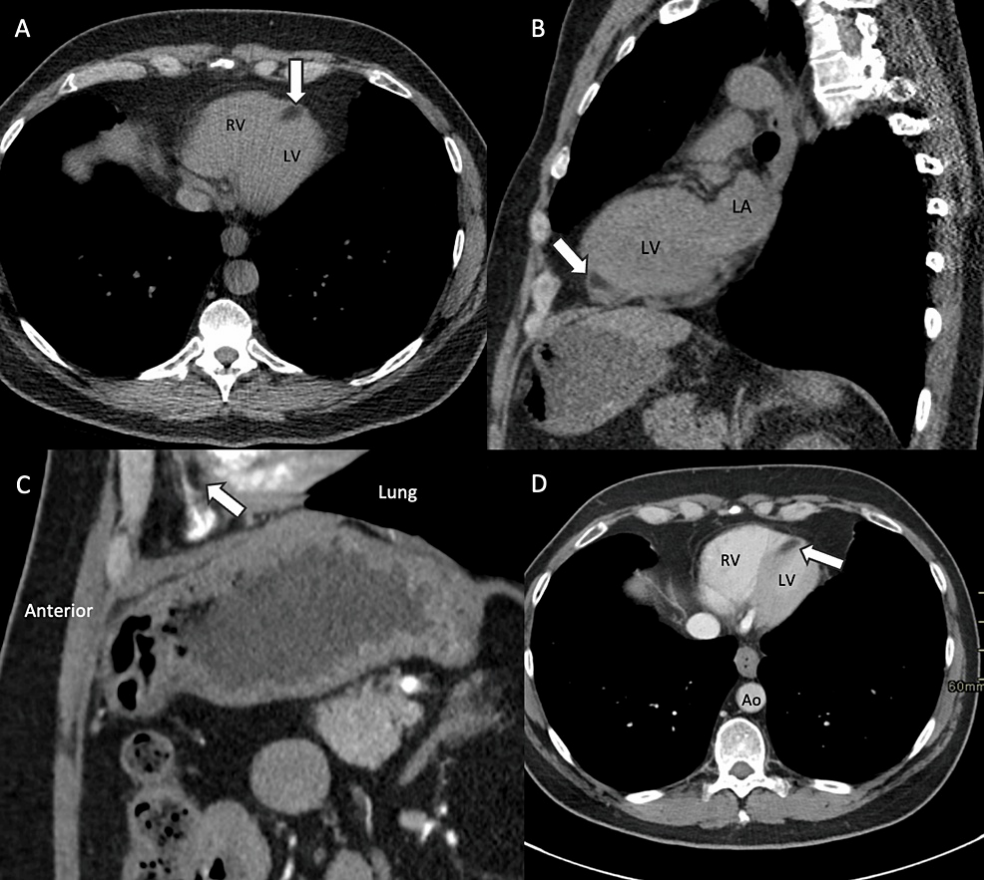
Axial (A) and sagittal (B) unenhanced computed tomography revealed a hypoattenuating fatty mass in the distal interventricular septum. Sagittal arterial phase (C) and axial venous phase (D) from a CT kidney six years prior better delineate the chambers of the heart and the position of the lipomatous mass within the septal wall. Axial arterial phase images did not reveal the lesion as clearly as sagittal images. RV: right ventricle; LA: left atrium; LV: left ventricle; Ao: aorta.
